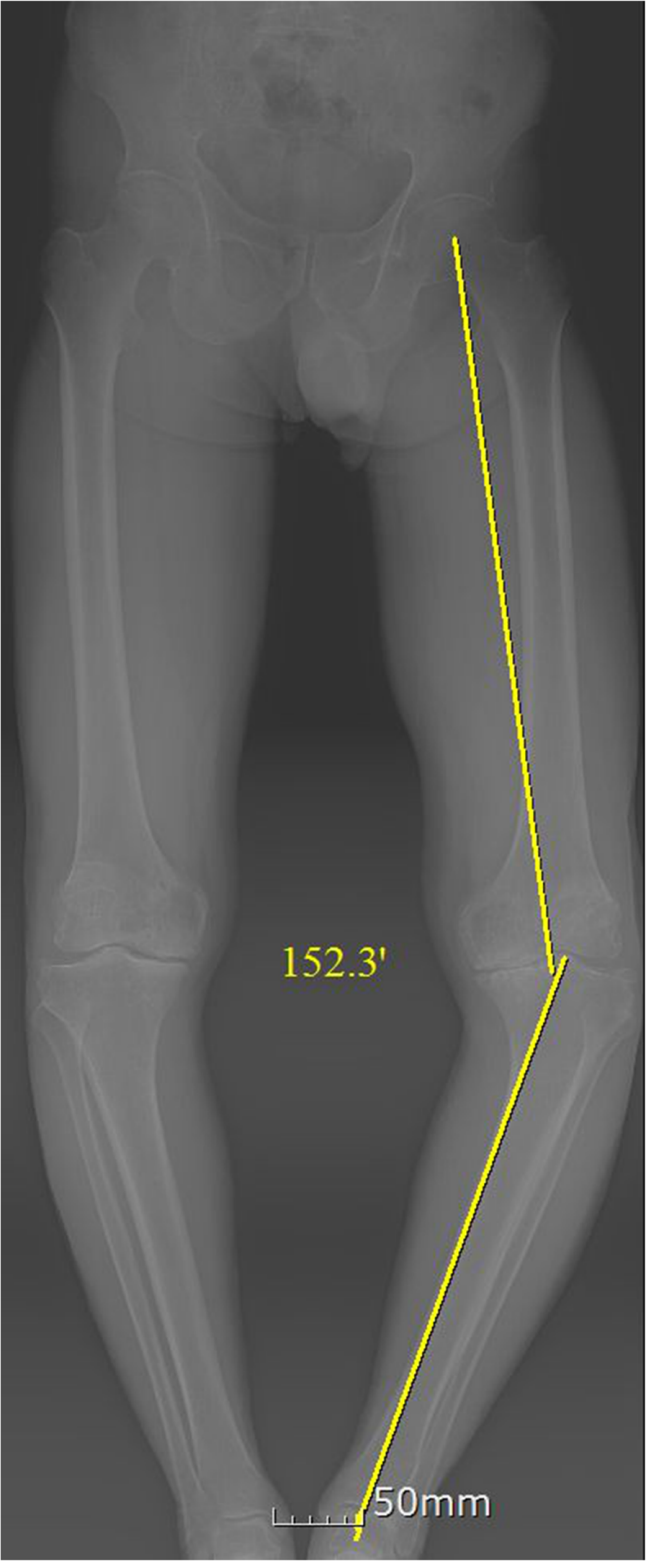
Preoperative long-standing anteroposterior radiograph of a patient with severe varus knee and marked medial bone loss
